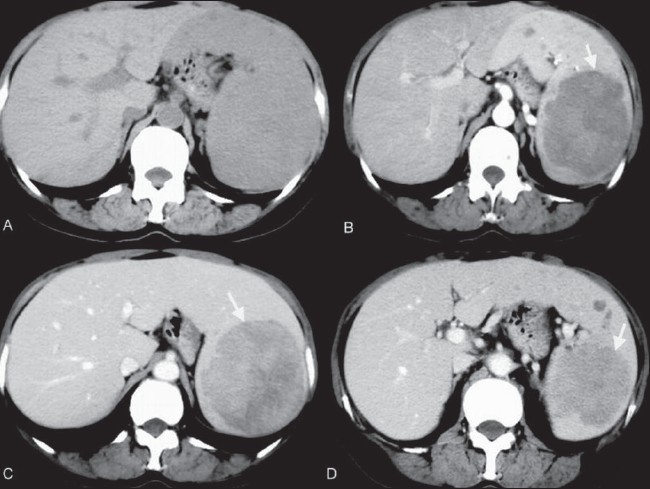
LCA of spleen. Case 1. CT scan. An axial nonenhanced image (A) shows no splenic mass. The arterial (B) and portal venous (C) images show a large, solitary, low-density lesion (arrow), the mass (arrow) becoming isodense to surrounding enhancing normal splenic tissue on the delayed phase image (D)
